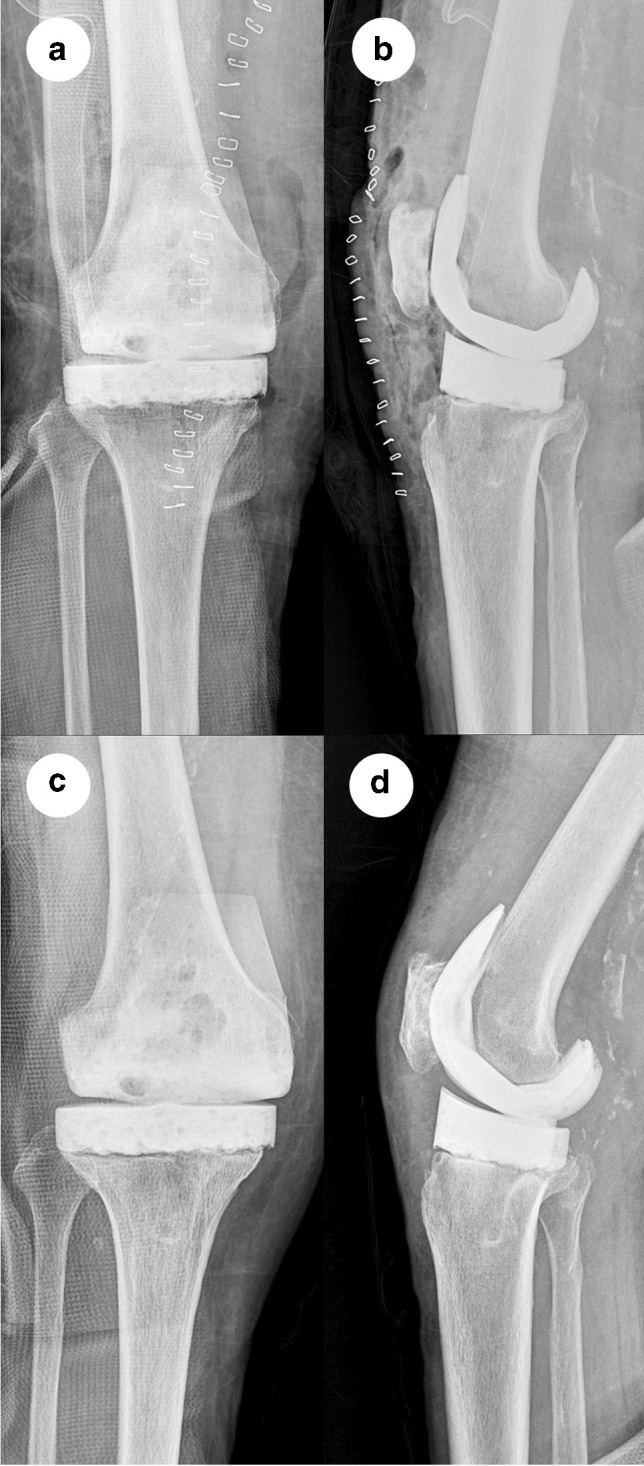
A 74-year-old woman with infected right knee prosthesis. Anteroposterior (a) and lateral (b) radiographs of the right knee after explanting the infected prosthesis and implanting the articulating cement spacer with spikes. Anteroposterior (c) and lateral (d) radiographs at 2 weeks prior to revision total knee arthroplasty. The interval changes in the tilting angle and mediolateral translation distance were 0.7° and 0 mm, respectively
